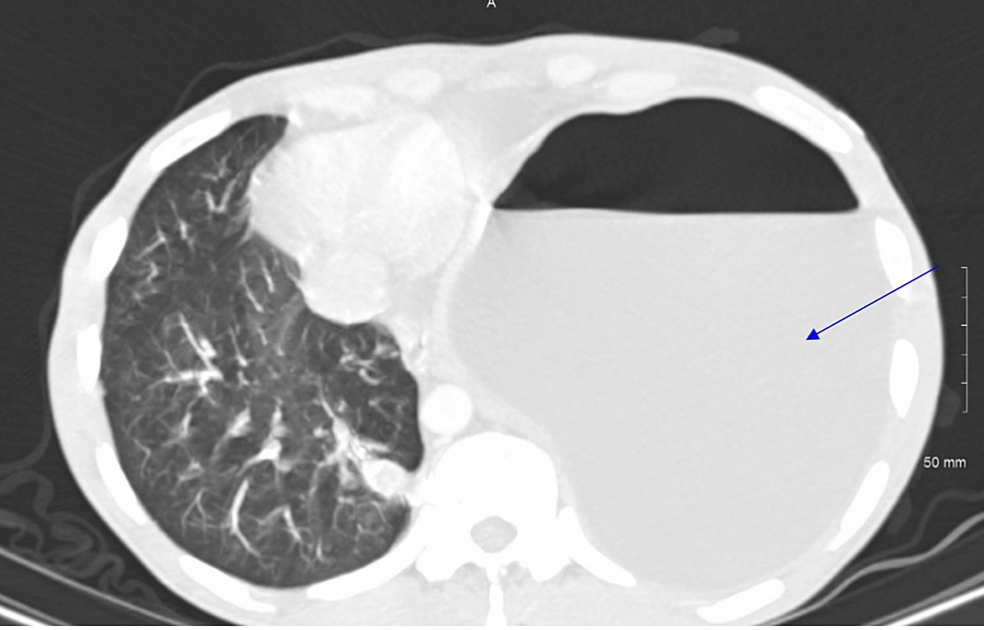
CT scan (axial) Axial CT scan shows a large air-fluid level (blue arrow) is seen in the left hemithorax with associated cardiac and mediastinal shift to the right.
